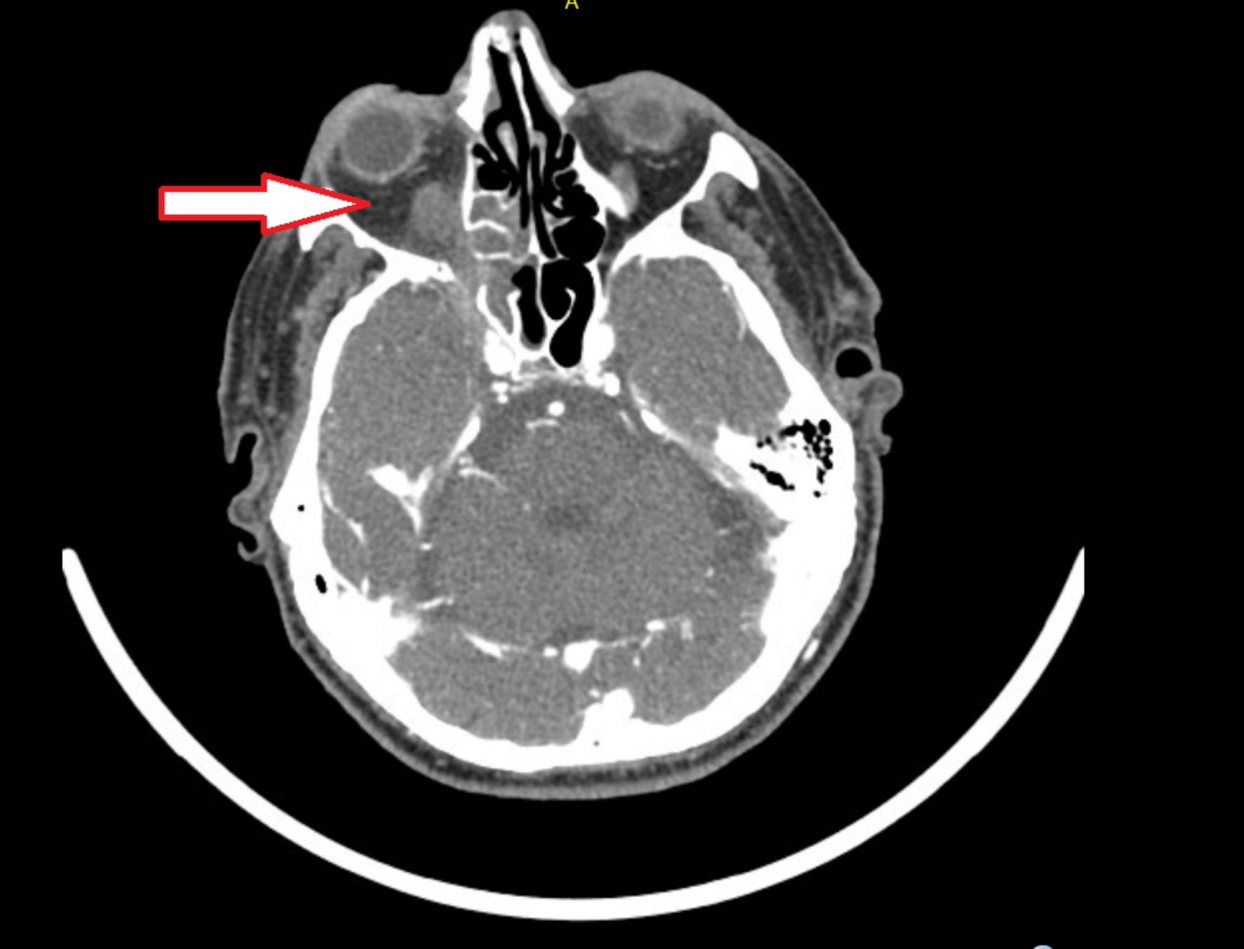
CT head concerning for invasive fungal sinusitis with abnormal soft tissue infiltrating the orbital apex (arrow).
